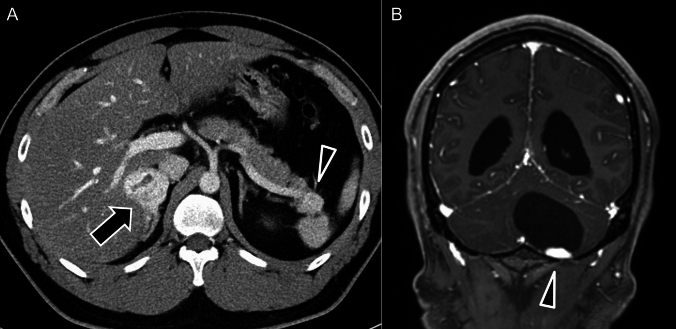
Pheochromocytoma related to von Hippel–Lindau disease. A Enhanced computed tomography showing a homogeneously enhancing mass in the right-adrenal gland (black arrow), consistent with pheochromocytoma, and a mass in the tail of the pancreas (arrowhead), suggestive of a pancreatic neuroendocrine tumor. B Gadolinium-enhanced T1-weighted magnetic resonance imaging (gadolinium-T1-weighted imaging) showing a cystic tumor with an intensely enhancing mural nodule (arrowhead) in the left cerebellum, consistent with a hemangioblastoma. These findings suggest von Hippel–Lindau disease with the characteristic involvement of pheochromocytoma, pancreatic neuroendocrine tumor, and cerebellar hemangioblastoma.
